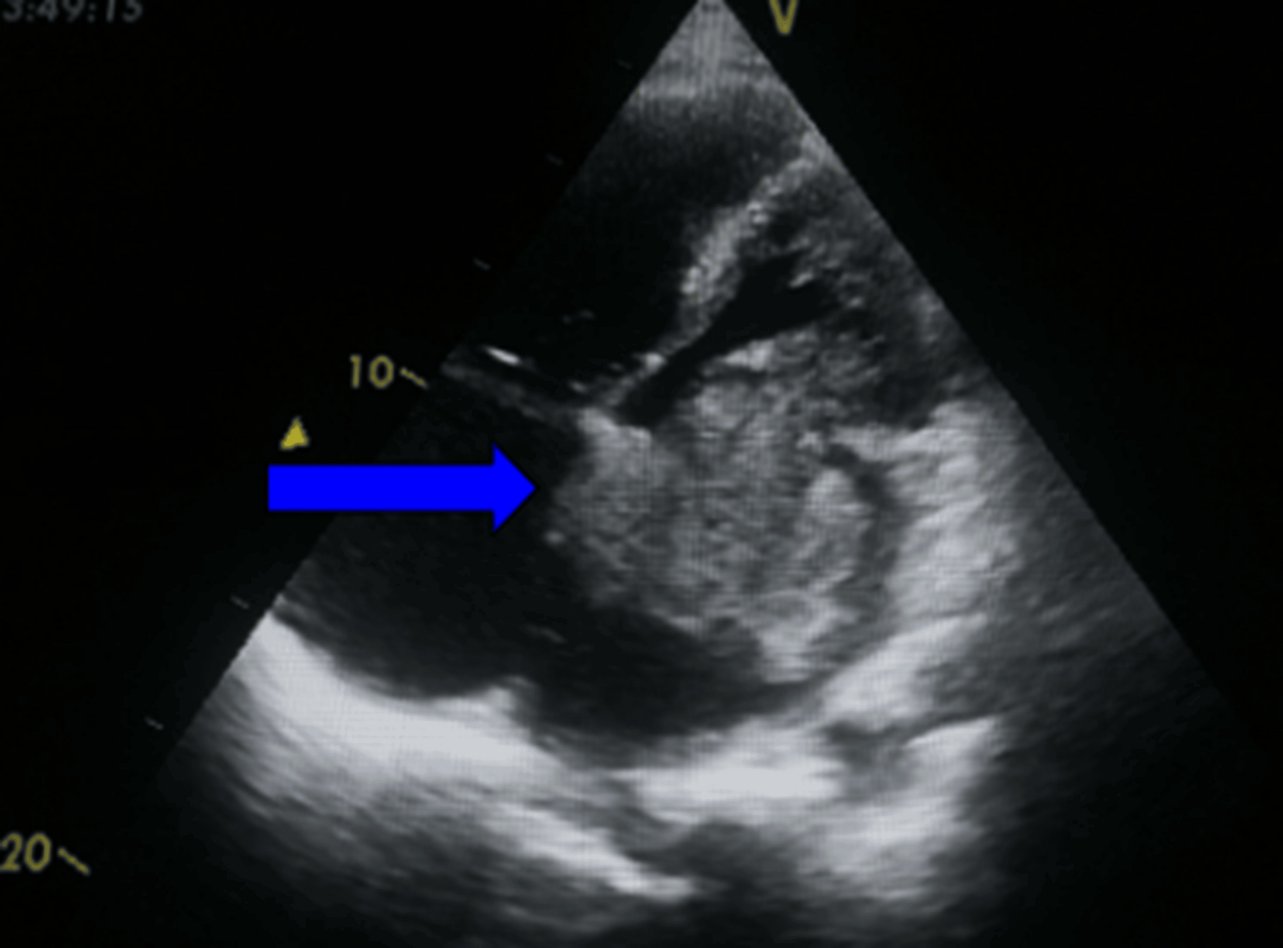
Transthoracic echocardiography (apical four-chamber view) showing a pedunculated mass attached to the interatrial septum that is obstructing the mitral valve during diastole (arrow)
